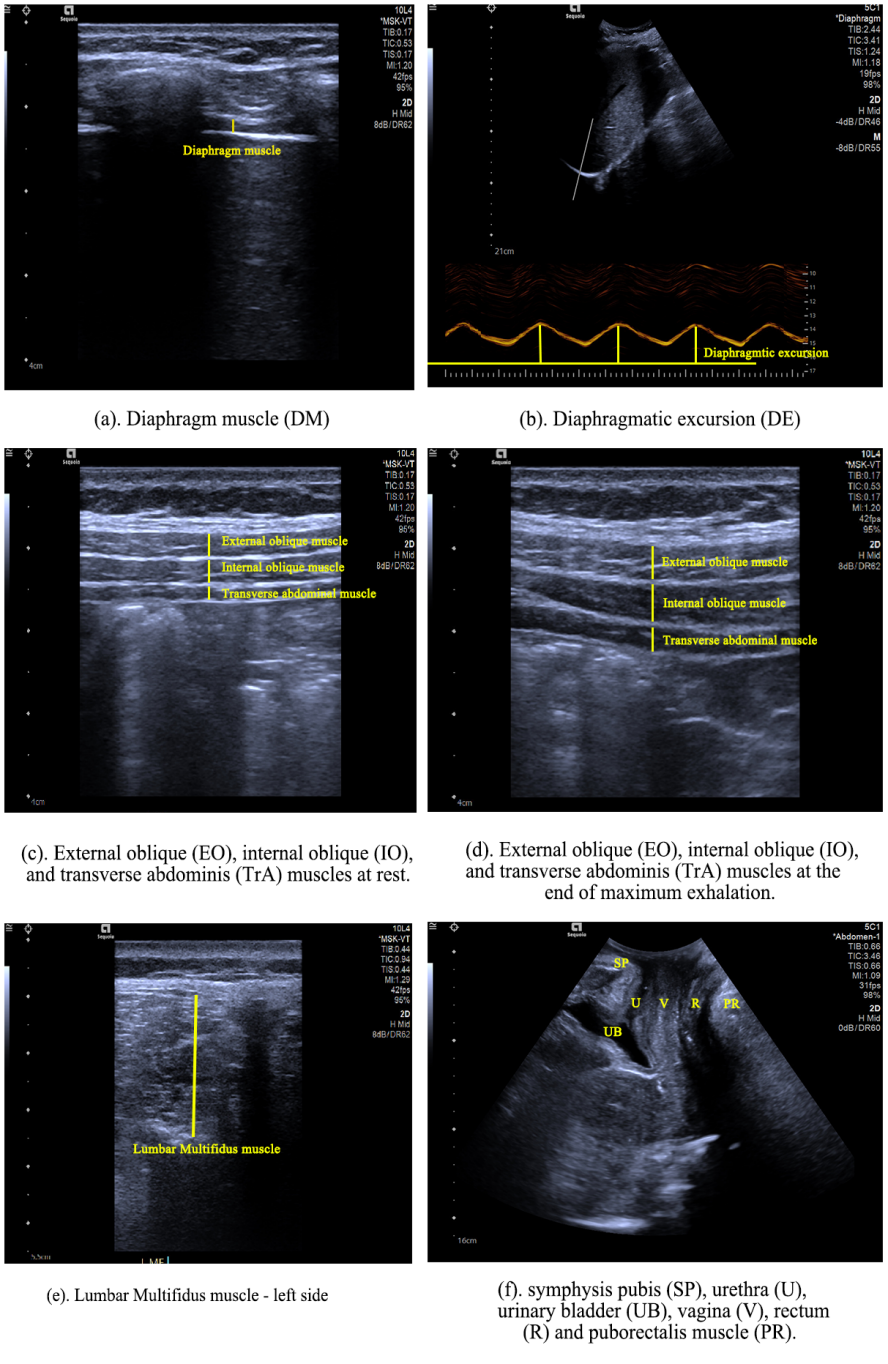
(A–F) Ultrasonographic images. (A) Diaphragm muscle (DM). (B) Diaphragmatic excursion (DE). (C) External oblique (EO), internal oblique (IO), and transverse abdominis (TrA) muscles at rest. (D) External oblique (EO), internal oblique (IO), and transverse abdominis (TrA) muscles at the end of maximum exhalation. (E) Lumbar Multifidis muscle (left side). (F) Symphysis pubis (SP), urethra (U), urinary bladder (UB), vagine (V), rectum (R) and puborectalis muscle (PR).
